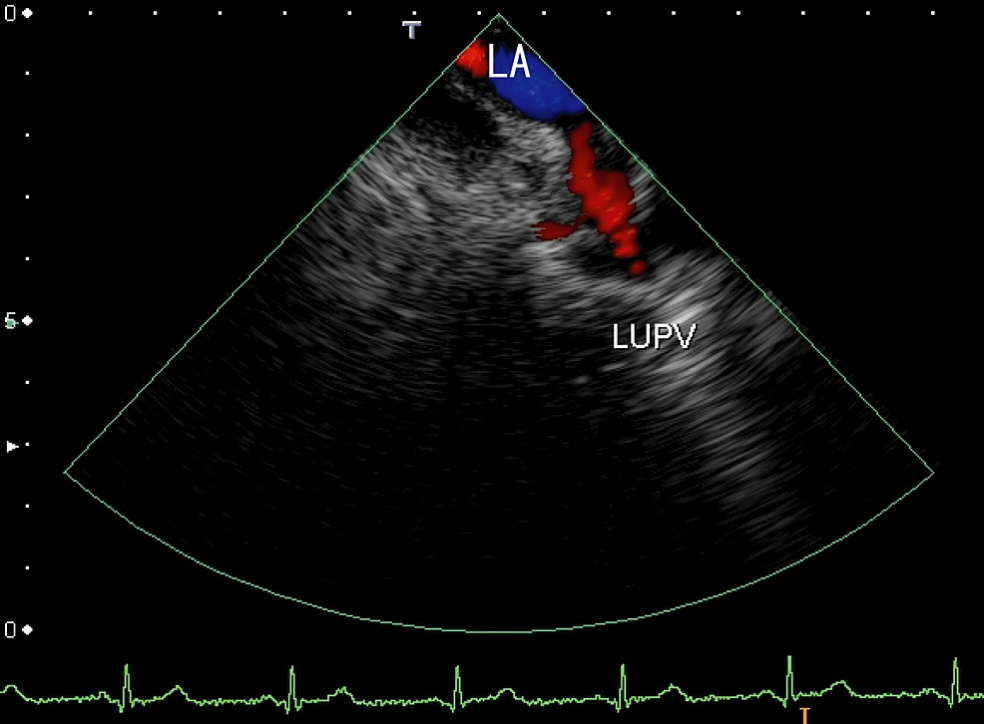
After the next nine months of anticoagulant treatment, TEE revealed no thrombi in the LA around the ostia of the LUPV LA: left atrium; LUPV: left upper pulmonary vein; TEE: transesophageal echocardiography
